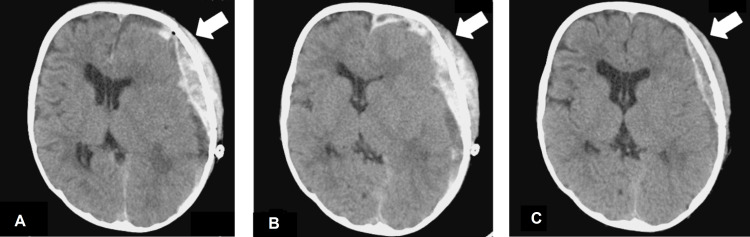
Head CT after resumption and re-discontinuation of anticoagulation (A) Head CT on day 7. Midline shift improved with a decrease in subdural hematoma; (B) Head CT on day 9. After anticoagulation therapy was resumed on postoperative day 7, the subdural hematoma gradually increased; (C) Head CT on day 22. Anticoagulation therapy was resumed on day 18, but no hemorrhagic complications were observed.
